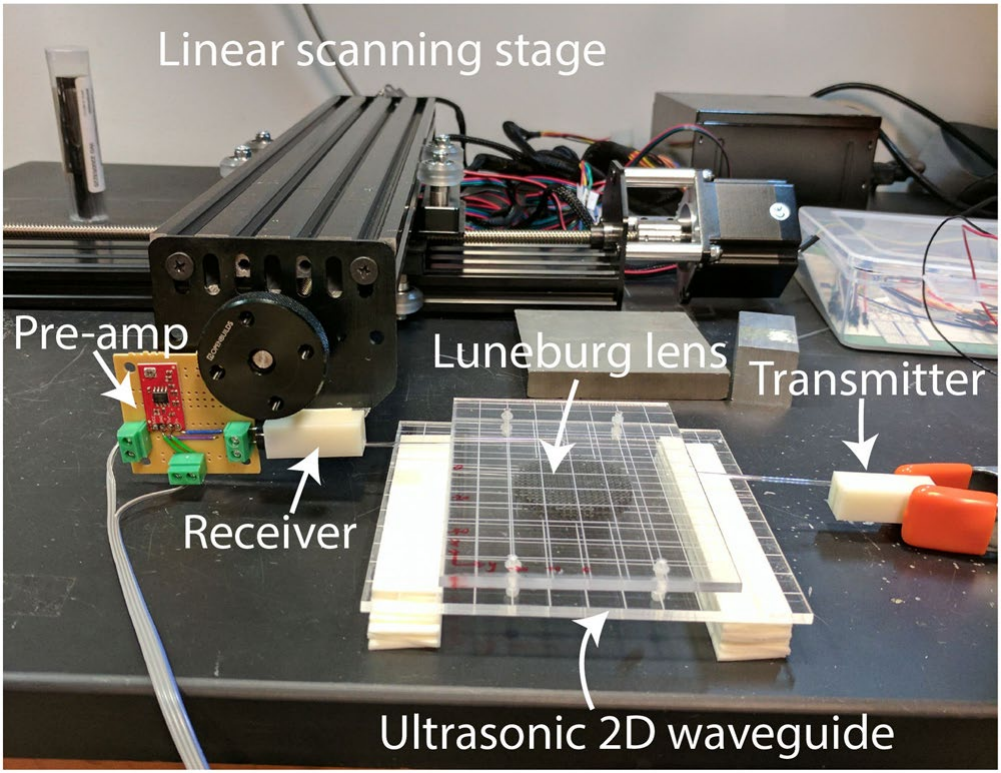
The experimental setup of the ultrasonic imaging experiment.
